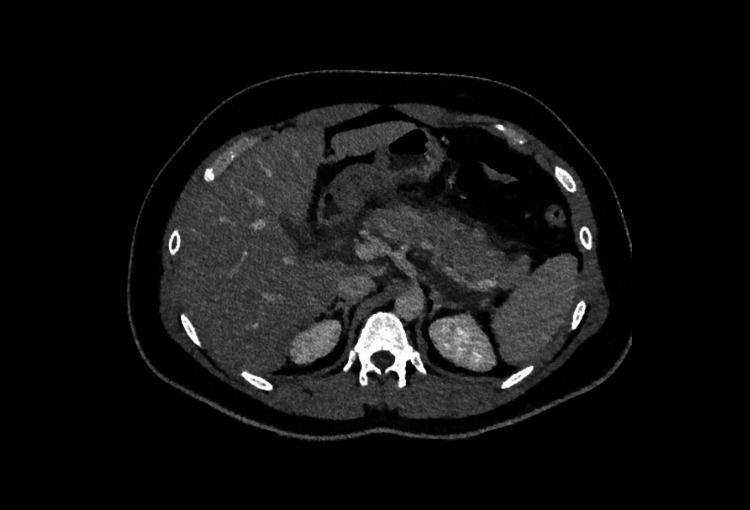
CT abdomen showing peri-pancreatic inflammatory stranding in keeping with acute severe pancreatitis, with no evidence of necrosis.
